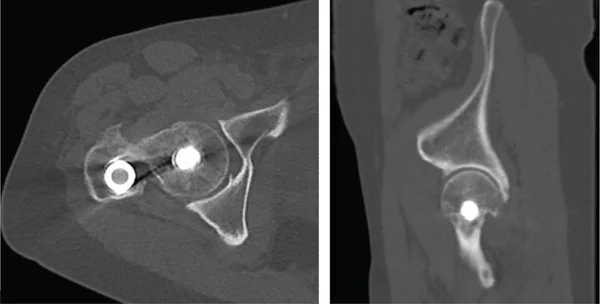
Axial and sagittal CT slices of right hip 9 days post subsequent injury.
